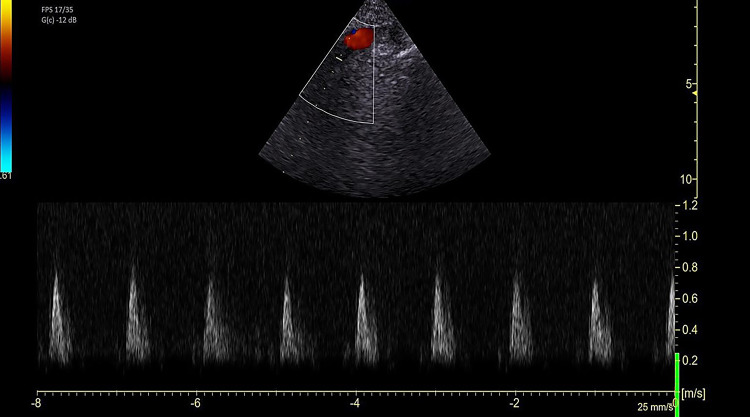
Example of peak velocity of aortic blood flow (V_peak_) sampled at the ascending aorta site. Images are taken with the aid of color doppler to recognize blood flow and ensure the best alignment
